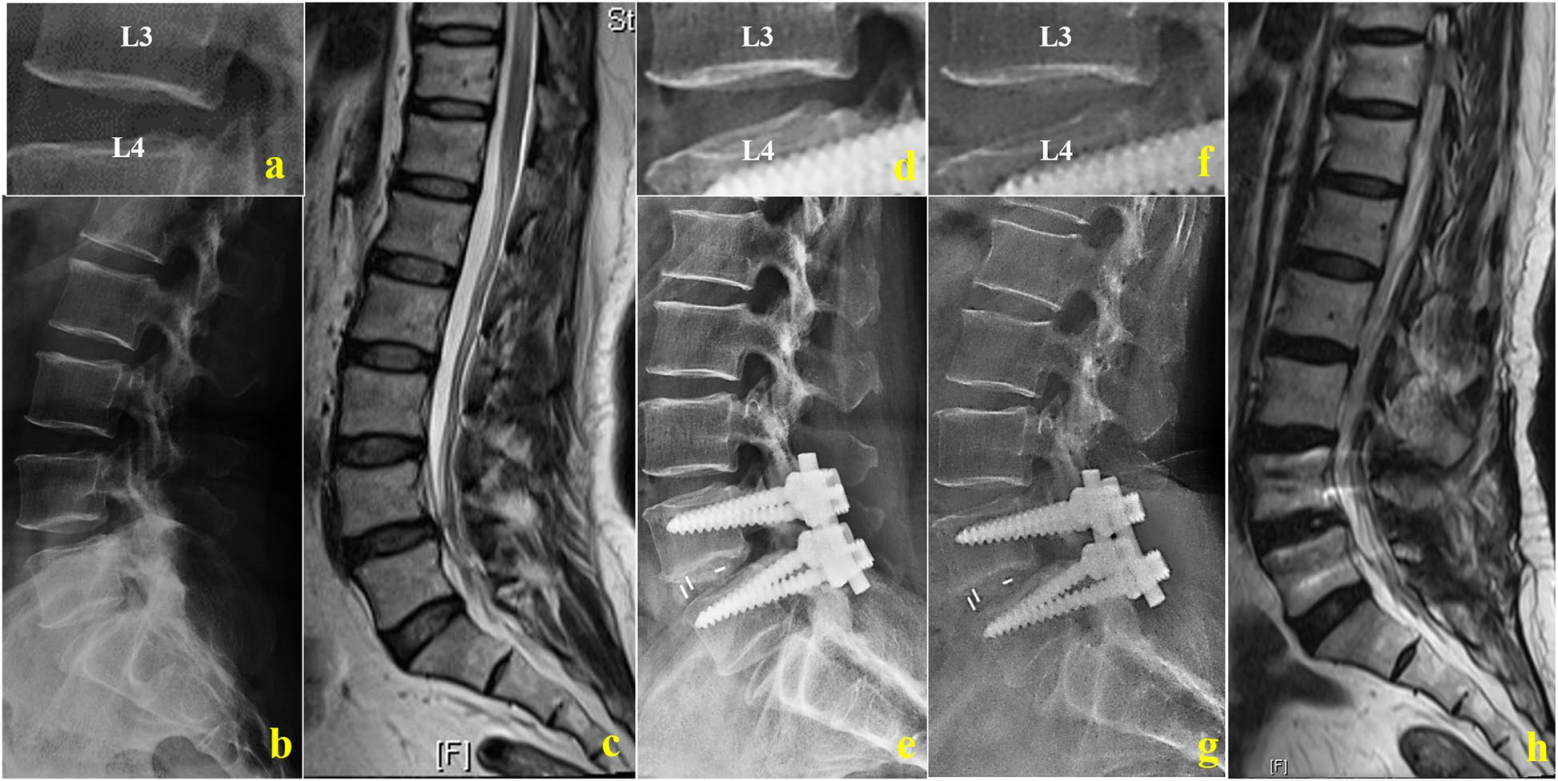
Radiological studies of a 76-year-old woman with lumbar spinal stenosis after PS-PLIF. a–c Preoperative plain radiographic image and preoperative sagittal magnetic resonance imaging (MRI) scan. Intervertebral space height (ISH): 13.03 mm, foraminal height (FH): 16.40 mm, foraminal width (FW): 9.12 mm, range of motion (ROM): 11.85°, lumbar lordosis (LL): 41.22°, sacral slope (SS): 31.95°, COBB 1: 13.90°, COBB 2: 21.40°, H 2/H 1: 2.61, and Pfirrmann classification of the cephalic lumbar intervertebral disk: grade I. d, e Postoperative plain radiographic images at 6-month follow-up. f–h Postoperative plain radiographic image and preoperative sagittal MRI scan at 38-month follow-up. ISH: 10.17 mm, FH: 14.66 mm, FW: 8.27 mm, ROM: 13.75°, LL: 56.12°, SS: 47.45°, COBB 1: 17.32°, COBB 2: 23.56°, H 2/H 1: 3.69, and Pfirrmann Classification: grade IV
